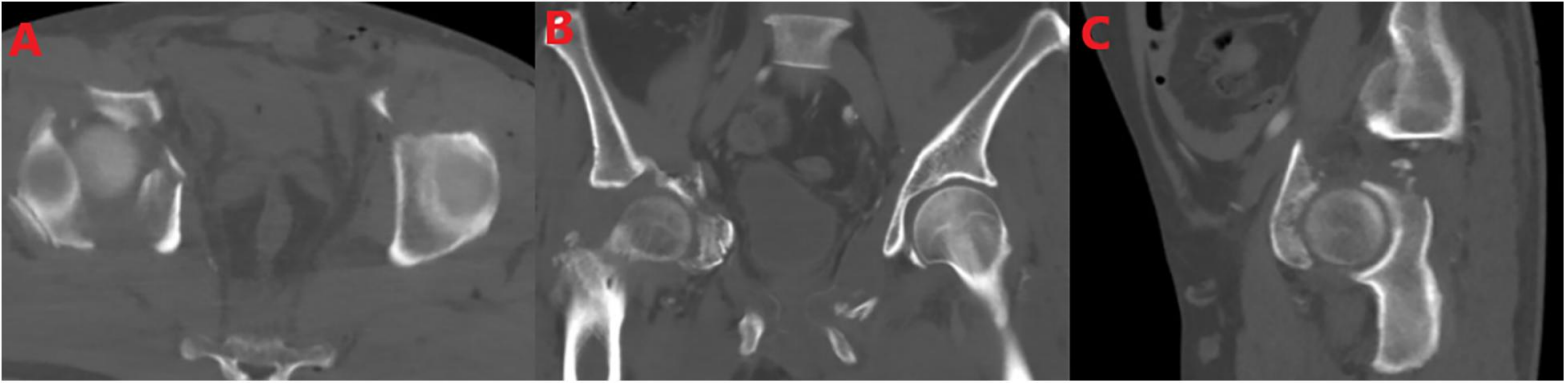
(A) Axial view, (B) coronal view, and (C) sagittal view. CT showed multiple fractures of the anterior column, posterior column, and quadrilateral plate of the right acetabulum, central dislocation of the right hip joint, and the femoral head displaced about 2.5 cm into the pelvic cavity.
